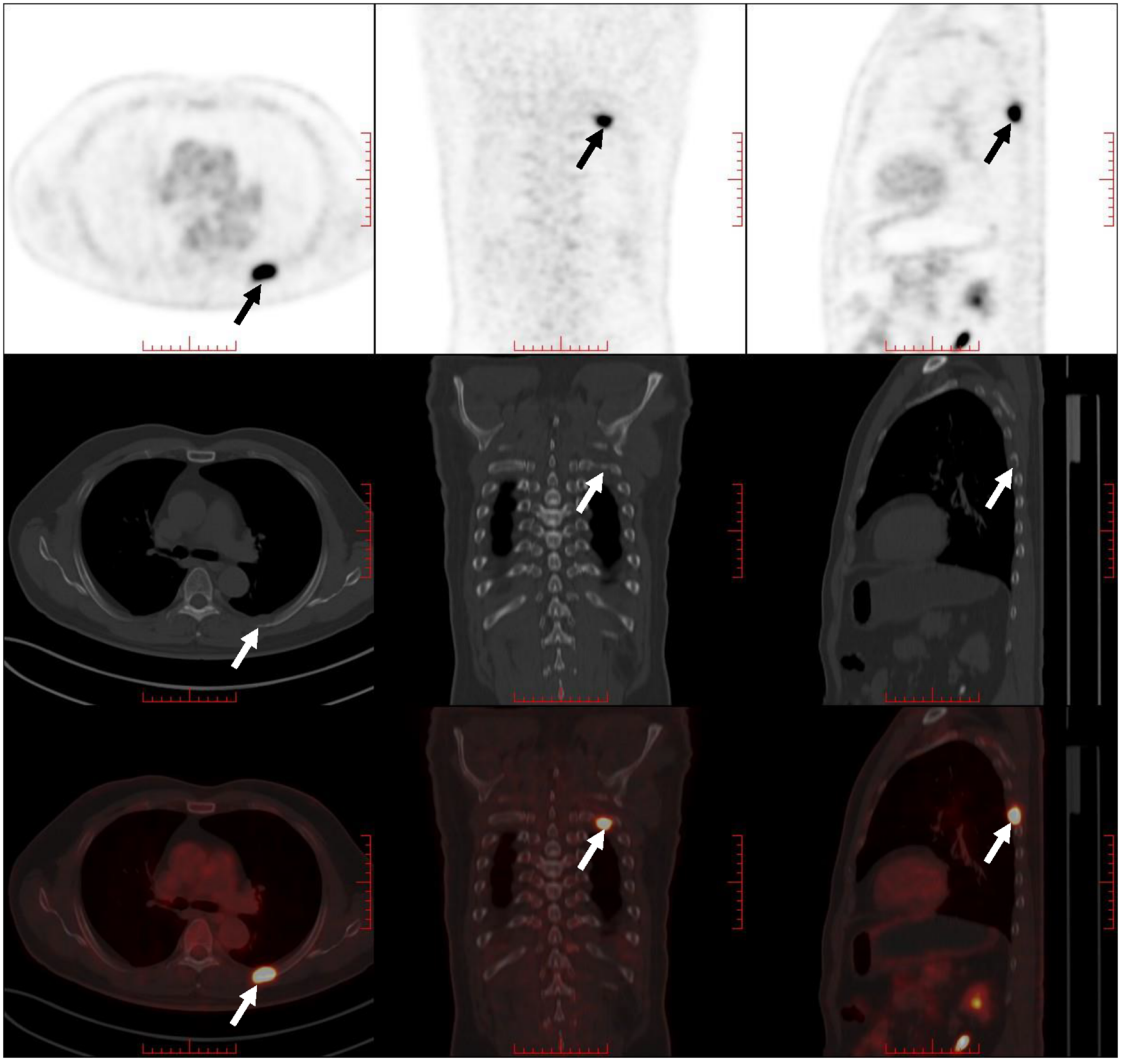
PET/CT image showed obvious osteolytic bone destruction and abnormal FDG uptake in the right fifth rib with local soft tissue mass formation (indicated by arrows).
